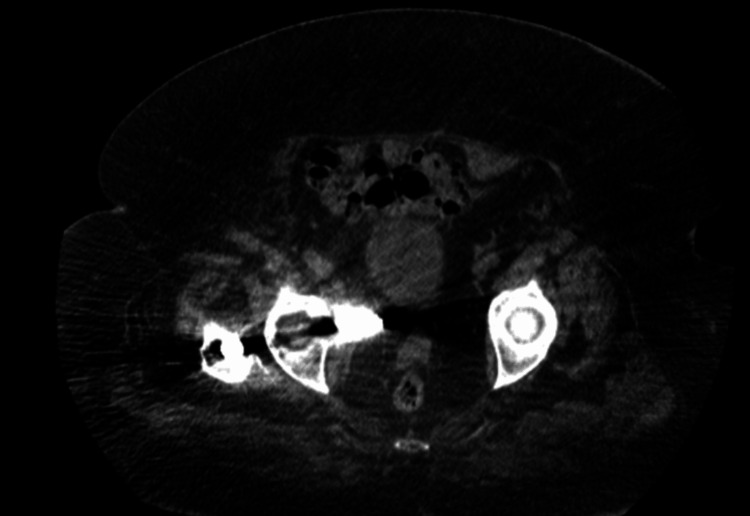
CT axial view demonstrating intrapelvic migration of the lag screw abutting against the rectum and vagina
